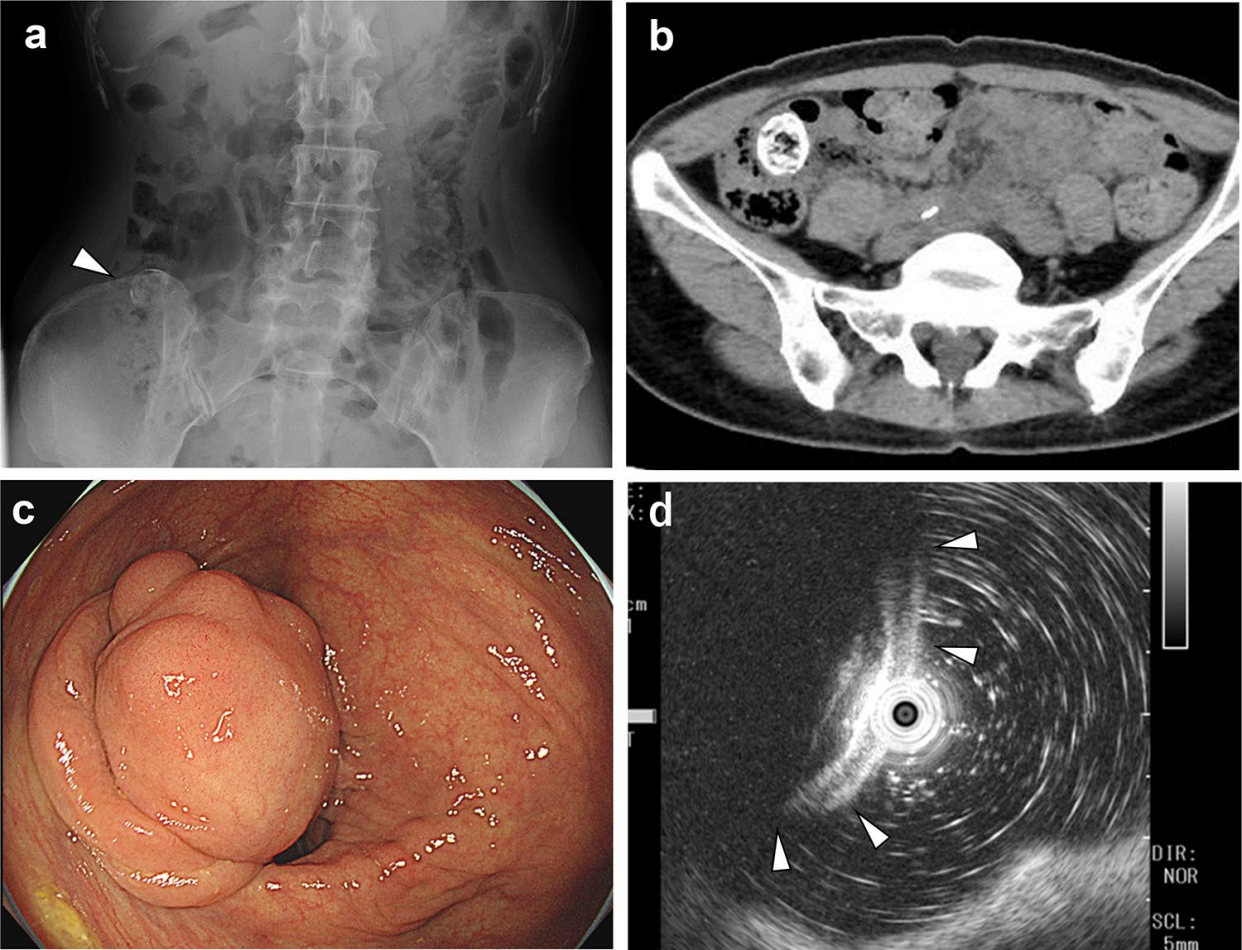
Preoperative imaging examination a Abdominal radiograph showing a lesion with calcifications in the right lower abdomen (white arrowhead). b Computed tomography image showing extensive tumor calcification. c A colonoscopy showed a 20-mm tumor on the ileocecal valve. The surface was covered by normal mucosa, and obstruction due to the tumor seemed likely. d EUS showed that the mucosa, submucosa, and muscularis layer were intact, and it was suspected that the tumor had originated from the third layer or a deeper location, but the sufficient observation was difficult due to calcification and other factors (the white arrowheads indicate the lesion covered by the normal colonic wall)
